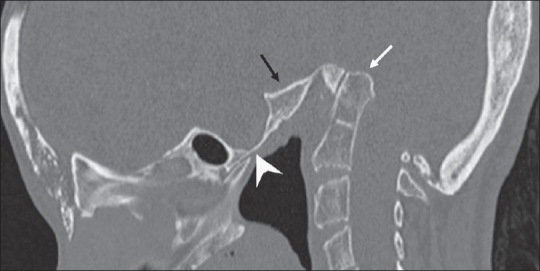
A sagittal CT scan sagittal images shows basilar invagination (arrow), enlarged pituitary fossa (arrowhead), and a deformed clivus (black arrow)
